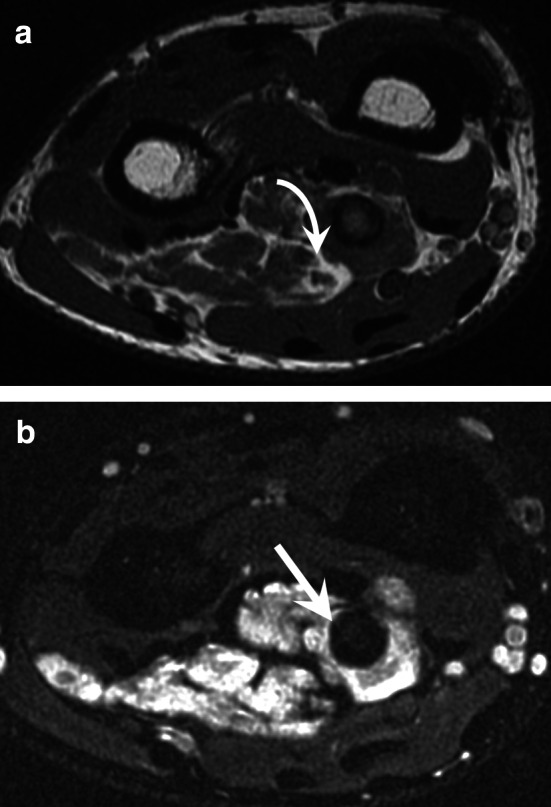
Intramuscular haemangioma in a 41-year-old man. a, b Axial T1-weighted (a) and T2-weighted (b) MR images showing a large mass infiltrating the flexor digitorum profundus muscle, composed of fatty tissue (curved arrow) and vascular lakes containing thrombi and calcifications (straight arrow)
